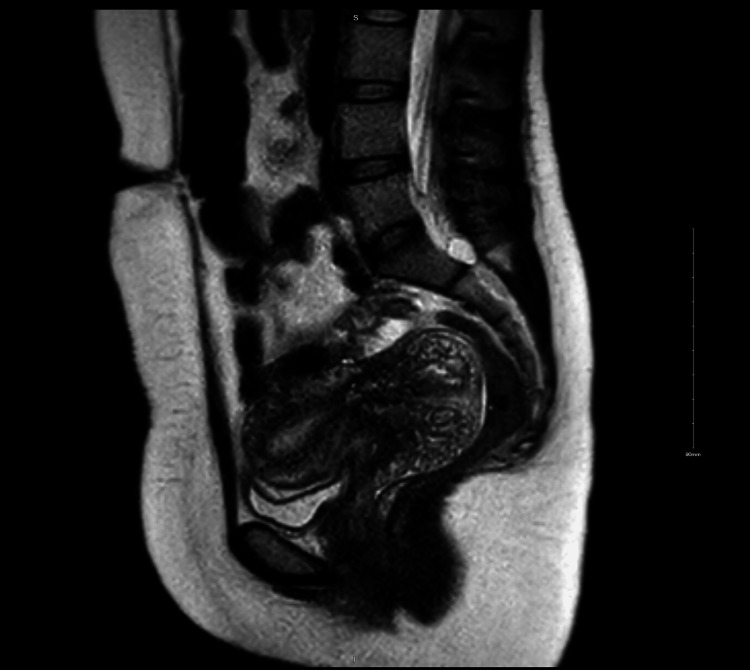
MRI T2-weighted image. Globular enlargement of the cervix containing multiple cystic spaces concerning adenoma malignum of the cervix, a variant of cervical mucinous adenocarcinoma
